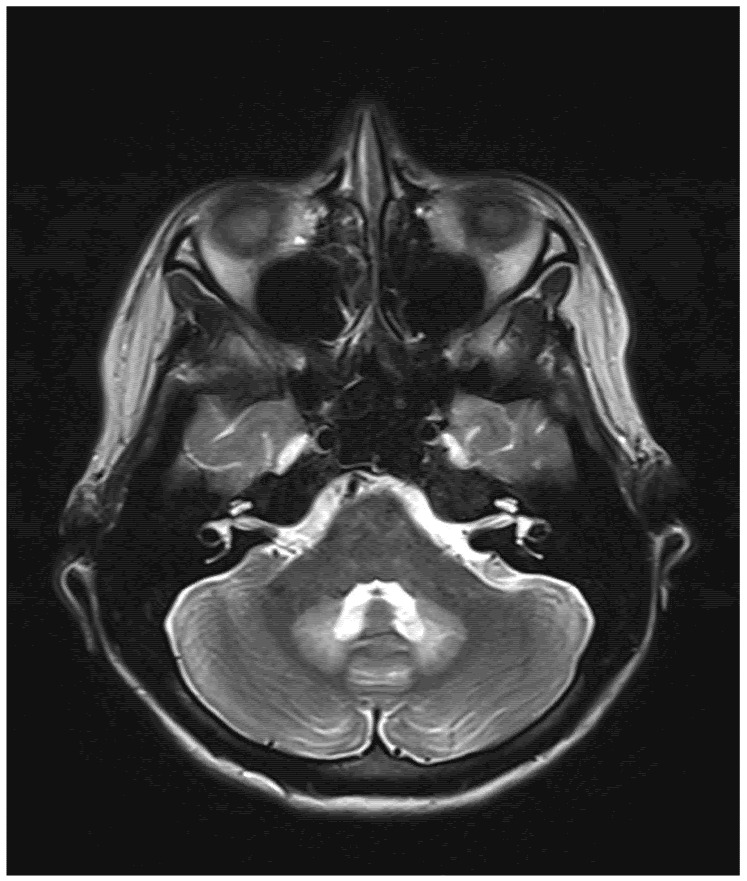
T2-weighted MRI axial view showing signal hyperintensity within dentate nuclei.
